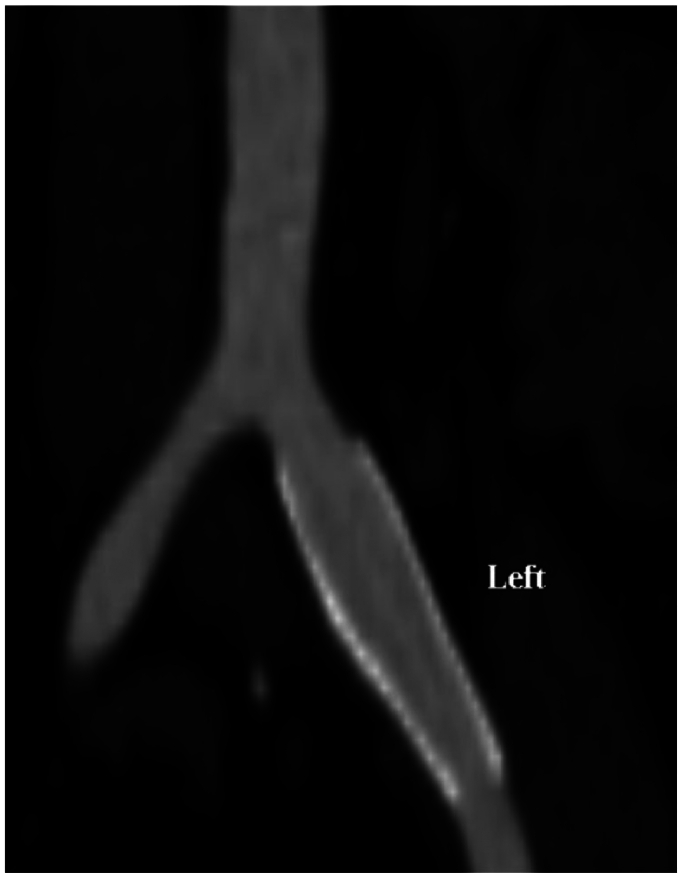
Computed tomography angiography (CTA) demonstrating successful iliac aneurysm repair with a stent graft by an outside hospital.
